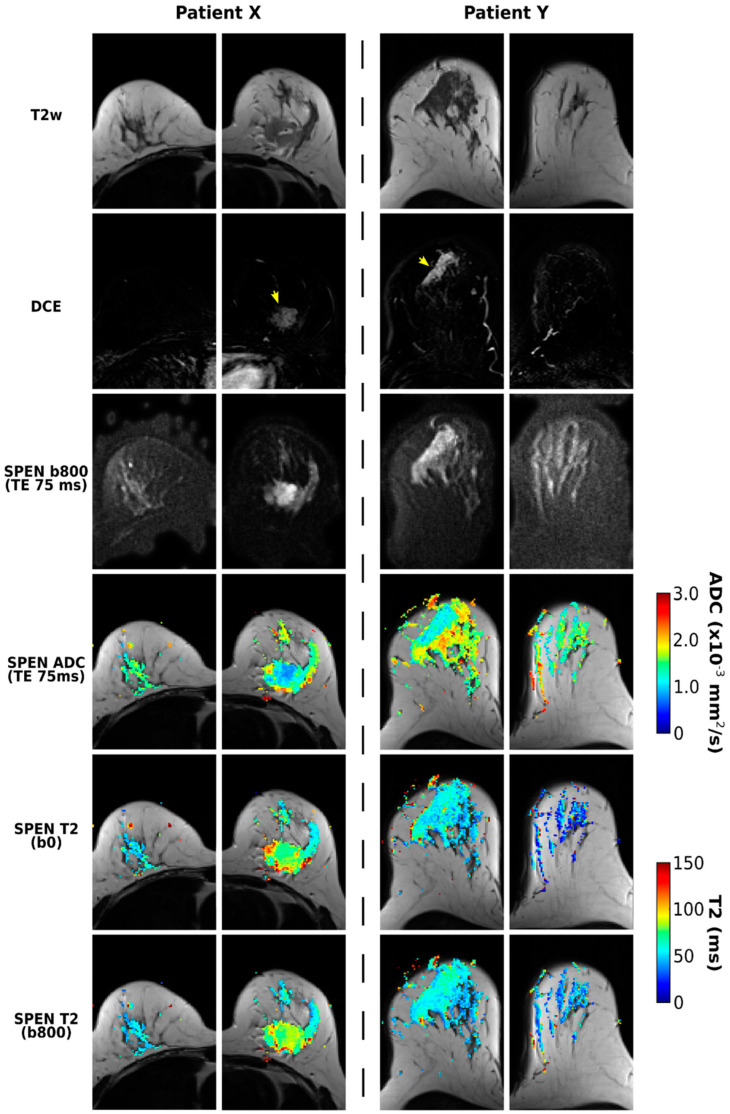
SPEN acquisitions for two patients (X and Y, corresponding to patients 12 and 7 in Table 1, respectively) diagnosed with breast carcinomas. Presented on the top rows are T2w, DCE, and DW images collected for TE = 75 ms, with the lesions labeled with yellow arrows. Shown on the lower rows are ADC maps derived from images acquired using TE = 75 ms, as well as T2 maps derived from b0 and b800 DWI data.
